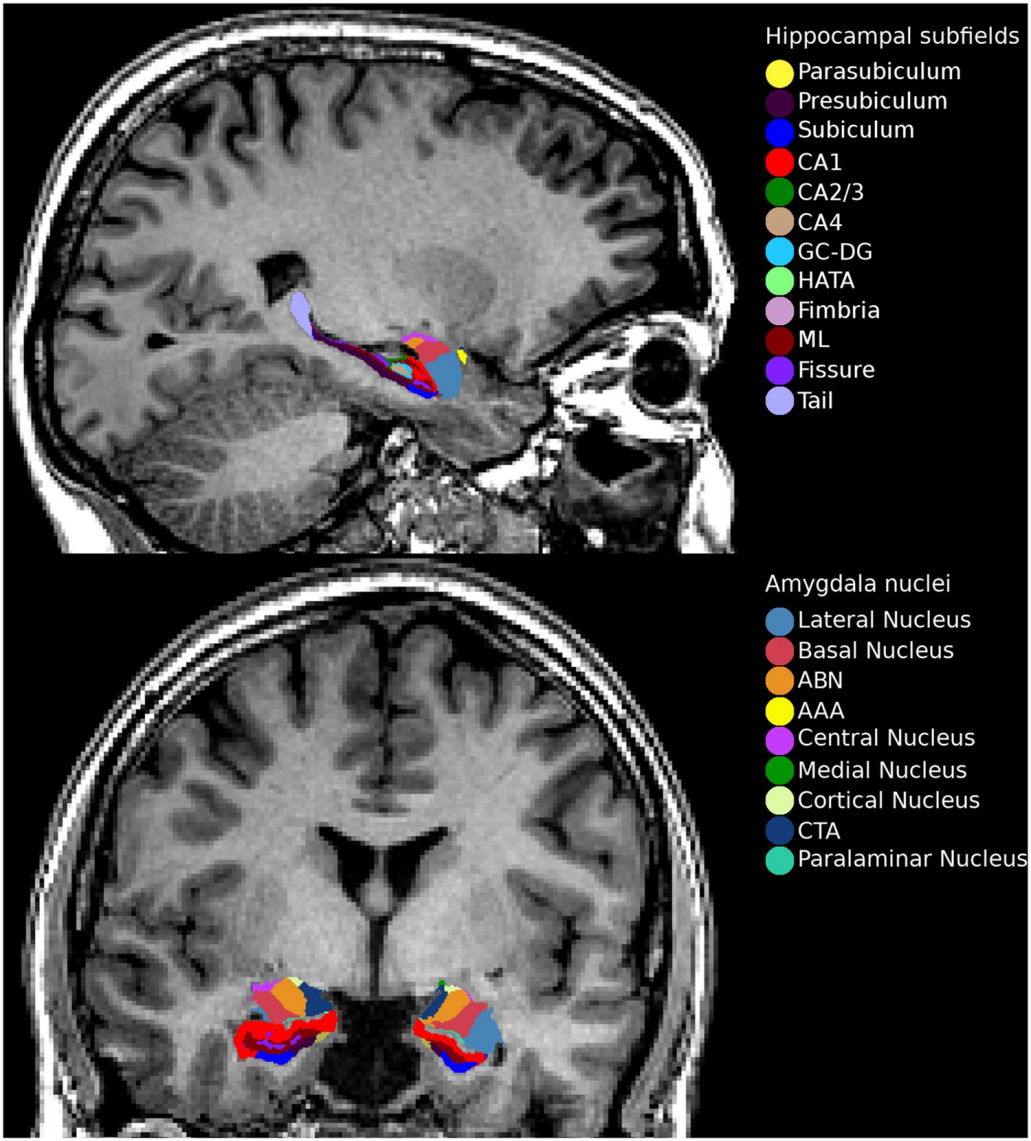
Sagittal and coronal view of the FreeSurfer 6.0 hippocampal subfield and amygdala nucleus segmentation. *CA* cornu ammonis, *GC-DG* granule cell layer of dentate gyrus, *HATA* hippocampal–amygdaloid transition area, *ML* molecular layer, *ABN* accessory basal nucleus, *AAA* anterior amygdaloid area, *CTA* cortico-amygdaloid transition area
